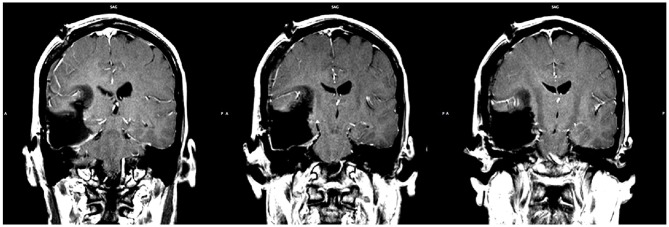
Post-operative MRI showing the extent of resection. Gross total resection was achieved (slice indices are approximately *y* = −28, *y* = −29, *y* = −30, from left to right respectively).
